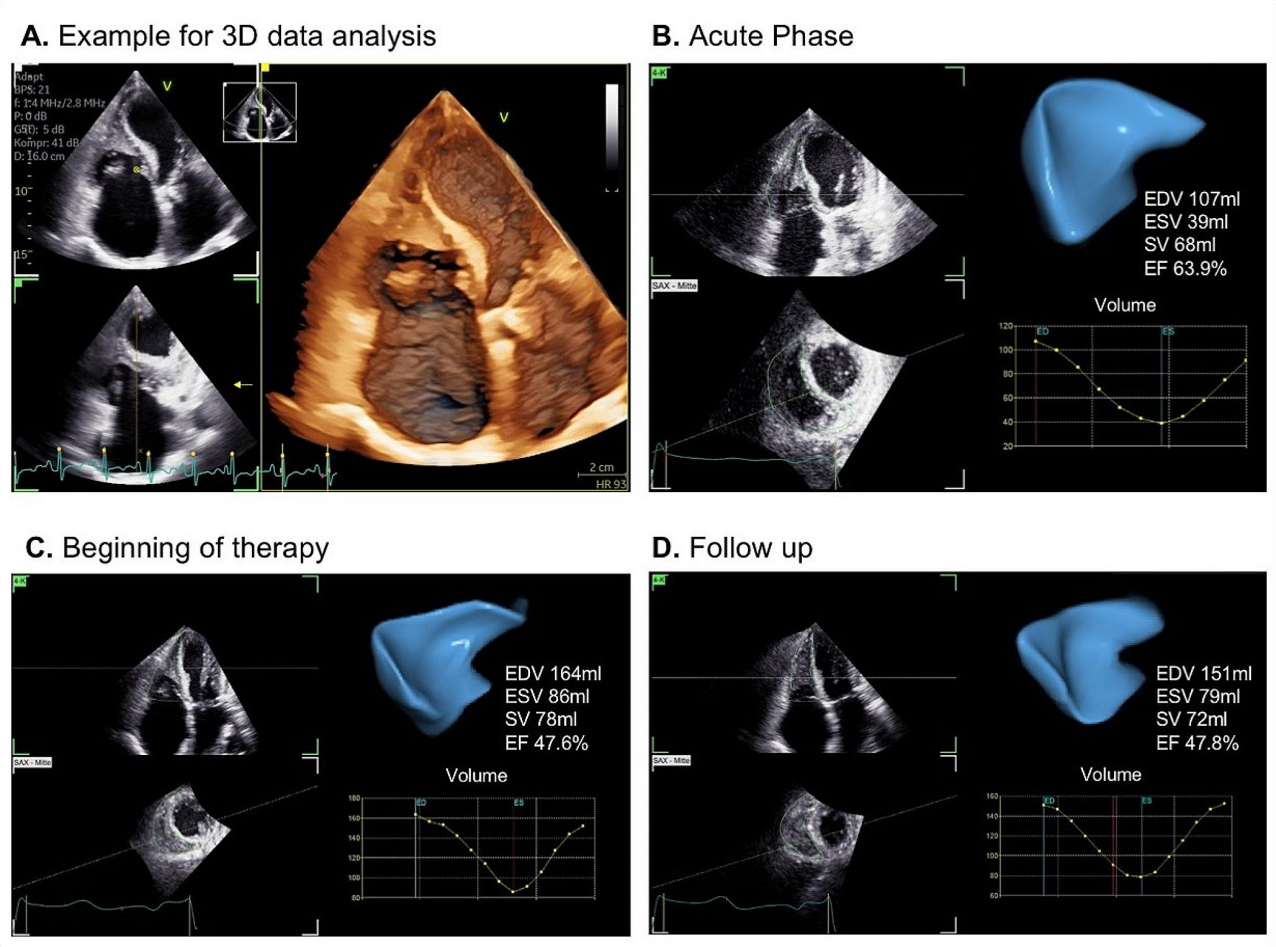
Right ventricular (RV) volume including thrombus formation of the right ventricular apex in a 3D data set at the acute stage of the Loeffler endocarditis (A), acute stage of inflammation (B), at early prednisolone therapy (C) as well as two weeks under ongoing immunosuppressive therapy (D). At baseline no 3D data acquisition was performed at routine echocardiography. RV focused 3D data sets were analyzed in the follow-ups
